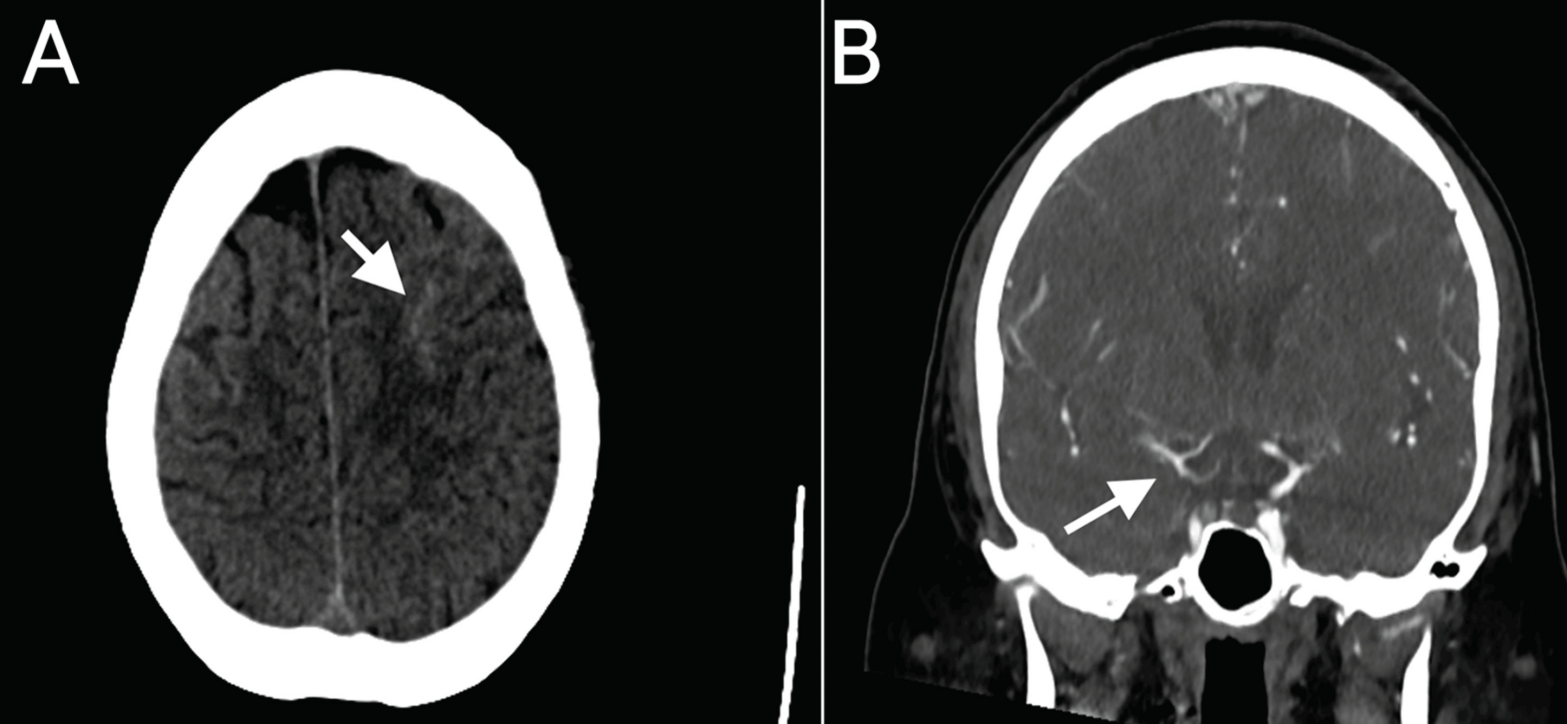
(A) Noncontrast axial CT of the head demonstrating left frontal subarachnoid hemorrhage (arrow). (B) Coronal CT angiogram demonstrating mild irregularity in the right middle cerebral artery/anterior cerebral artery (arrow) but no evidence of vessel occlusion. CT: computed tomography.
